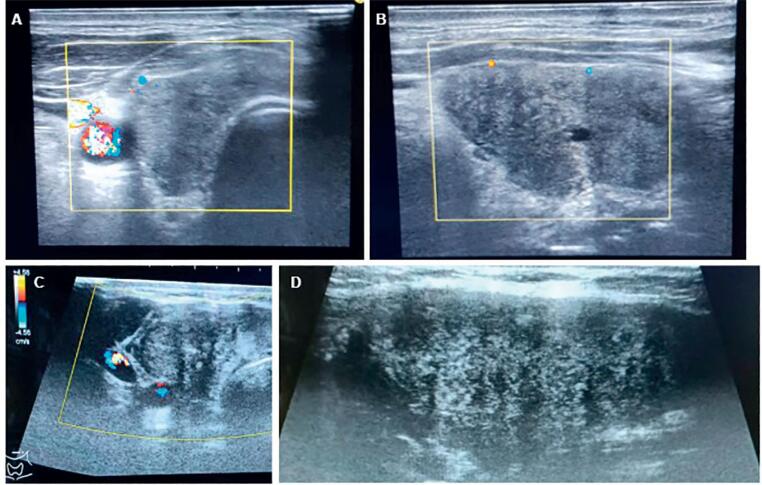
Thyroid ultrasonography (USG) images of the patients. Patchy hypoechoic areas with a lack of flow color on Doppler USG in the right lobe of thyroid gland in the transverse (
